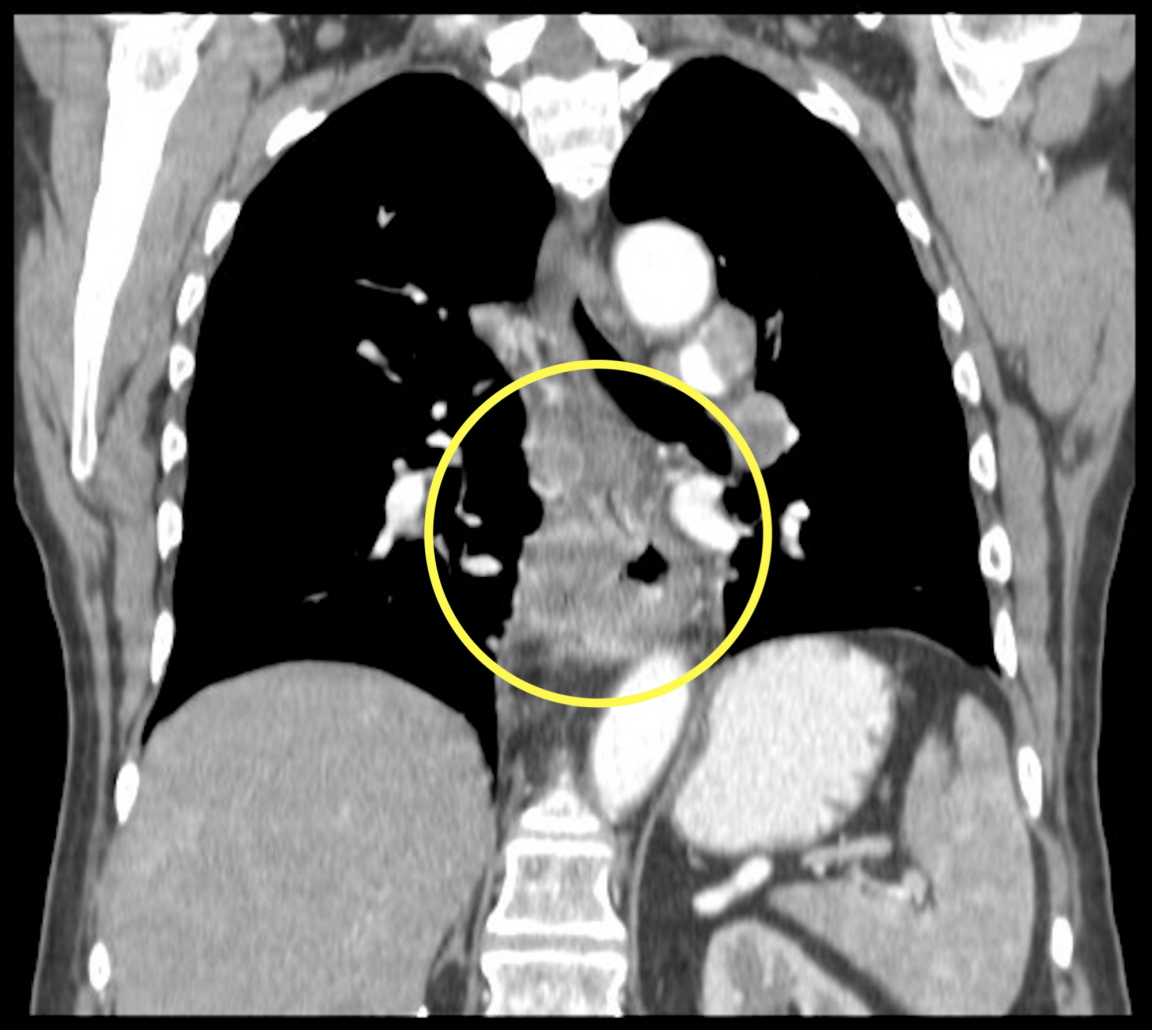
Coronal contrast enhanced computed tomography (CT) chest (soft tissue window) demonstrating a solid heterogeneously enhancing esophageal mass (yellow circle).
